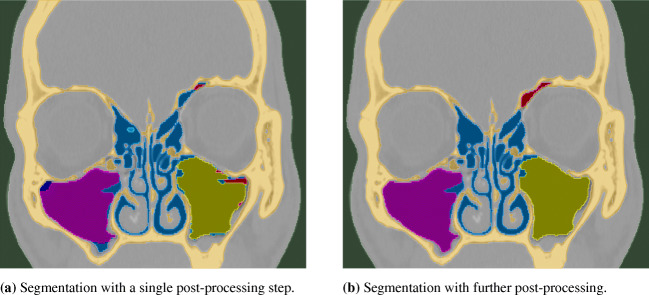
Multi-class CNN segmentation with and without post-processing for a single CT slice
